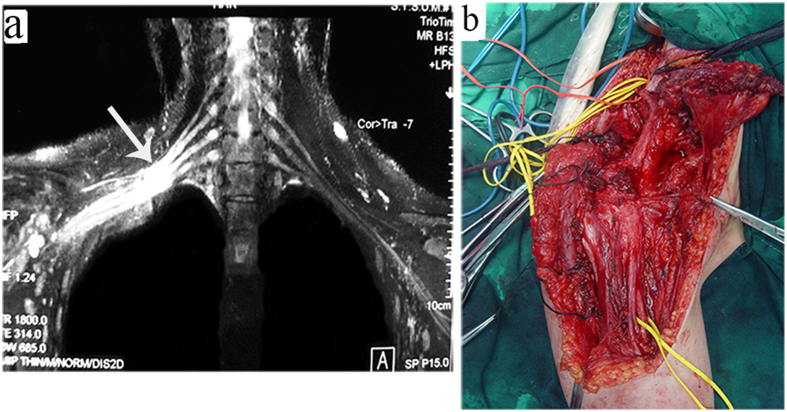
An 18-year-old female with a history of stretch injury by machine. (a) Coronal image shows disappearance of local nerve structure, replacement by scar tissue or hematoma, and neural appearance could not be observed on MRI at the level of the trunks and cords. (b) Accompanying clinical picture shows as figure a at the same level and surrounding scarring. a long segment of scar tissue surrounding the nerves and nerve defect no more than 20 cm.
